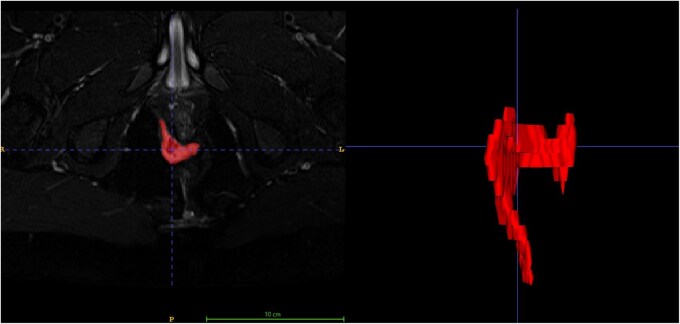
3D segmentation of posterior horseshoe using ITK-Snap. Left: Axial T2-weighted fat-saturated sequence demonstrating a posterior horseshoe abscess in a patient with complex pfCD. Right: Three-dimensional fistula reconstruction, shown from an anterior-posterior perspective of the same scan shown left generated in ITK-Snap following manual segmentation by a gastrointestinal radiologist.
